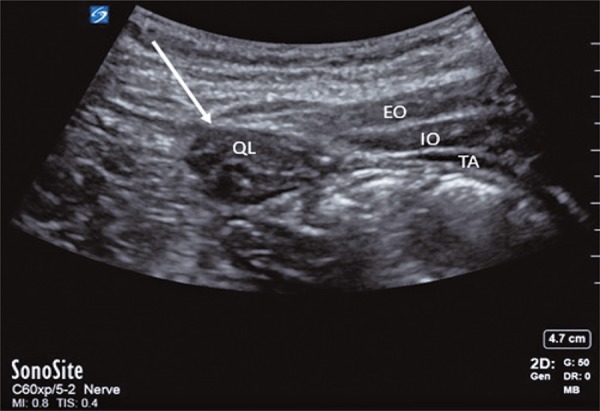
Ultrasound guided approach to posterior quadratus lumborum block. White arrow indicating posterior border of quadratus lumborum muscle
